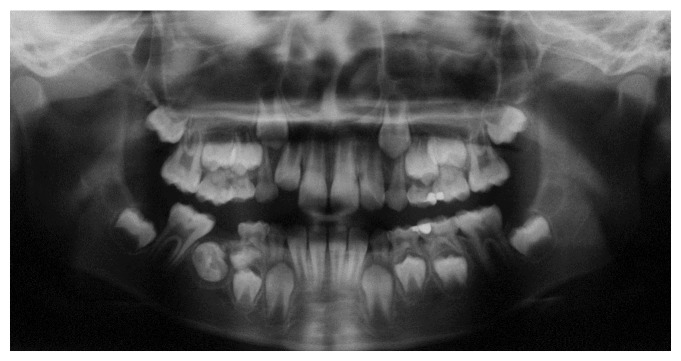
Initial panoramic radiograph: case  1. Complete impaction and mesiolingual inclination of the mandibular right second primary molar in the mandibular bone.
